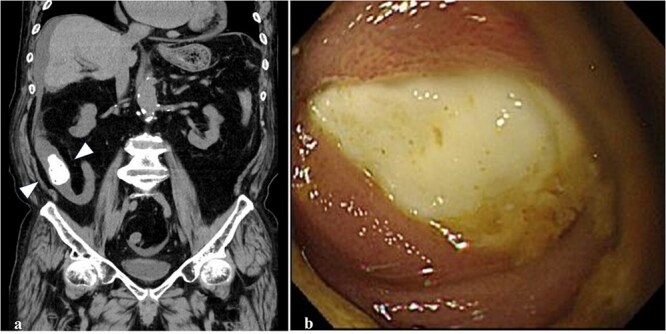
Abdominal CT and colonoscopy. (a) A highly dense mass with fine, low-density spots inside the lumen of the terminal ileum (arrowhead) on the colonal slice of plane CT. (b) The ileum lumen 20 cm proximal from the ileocecal valve was completely occupied by a whitish FB with a hard elasticity and smooth surface when visualized using a colonoscope.
